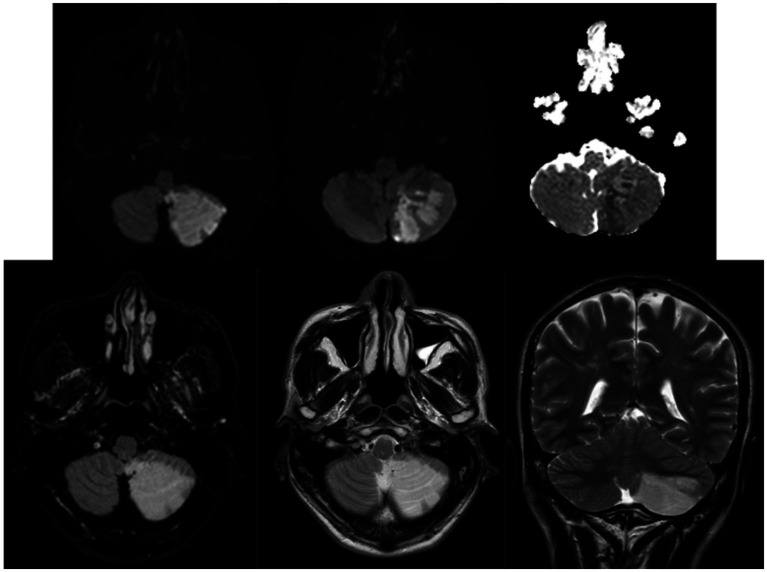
Case 2 MRI examination demonstrates the presence of an ischemic lesion in the left PICA area.
